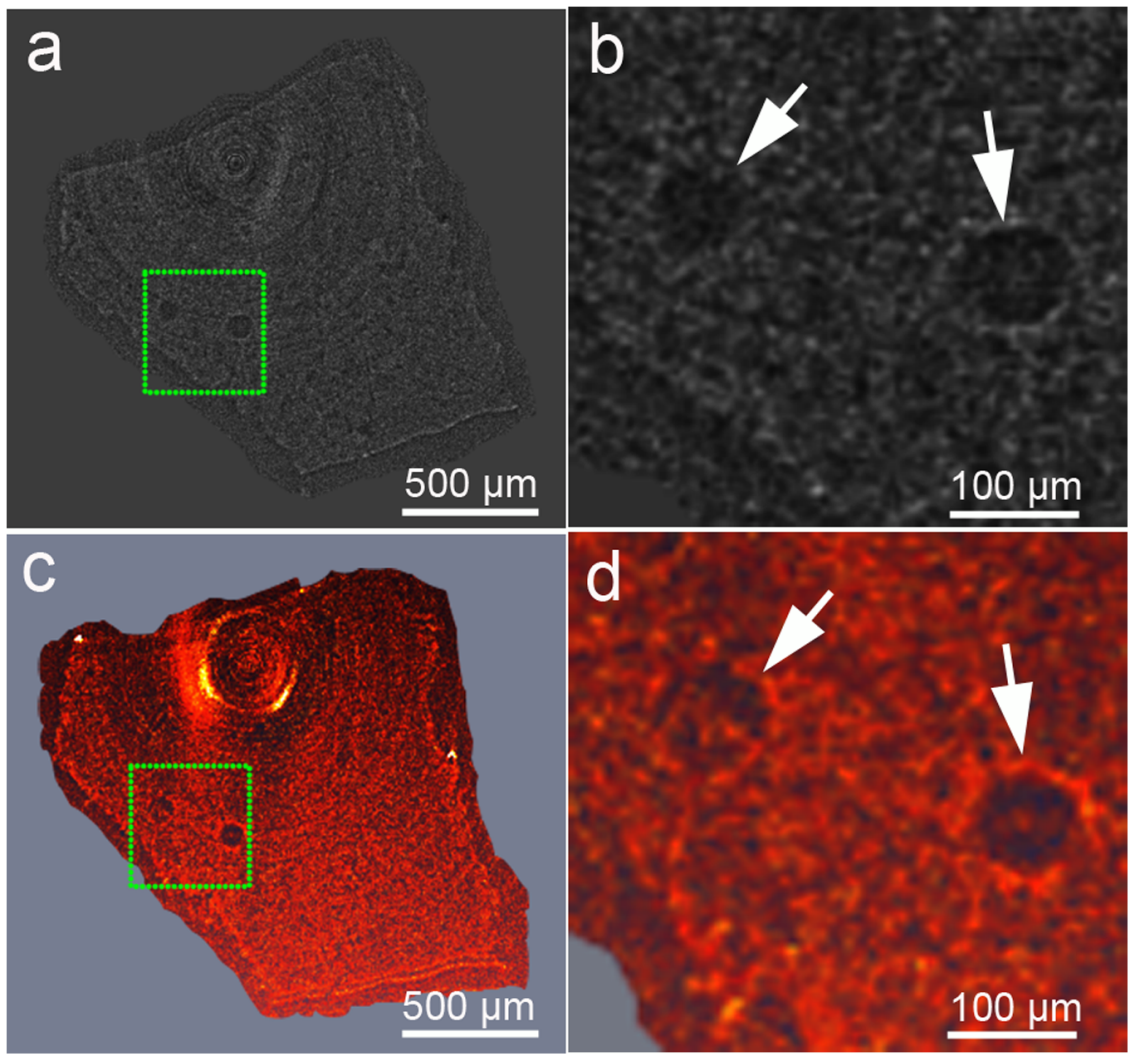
Phase contrast CT imaging of FCM-embolized non-dehydrated liver. Axial (a) and 3D (c) views of FCM in the liver. (b) and (d) are magnified images of the region in a dotted box in (a) and (c), respectively. Note that the phase contrast CT imaging clearly showed the radiolucent FCM in the liver tissues. Arrows indicate FCM. Images were obtained at the energy of 19 keV with the sample-to-detector distance of 60 cm. The pixel size was 3.7 µm×3.7 µm; the exposure time was 1 s.
